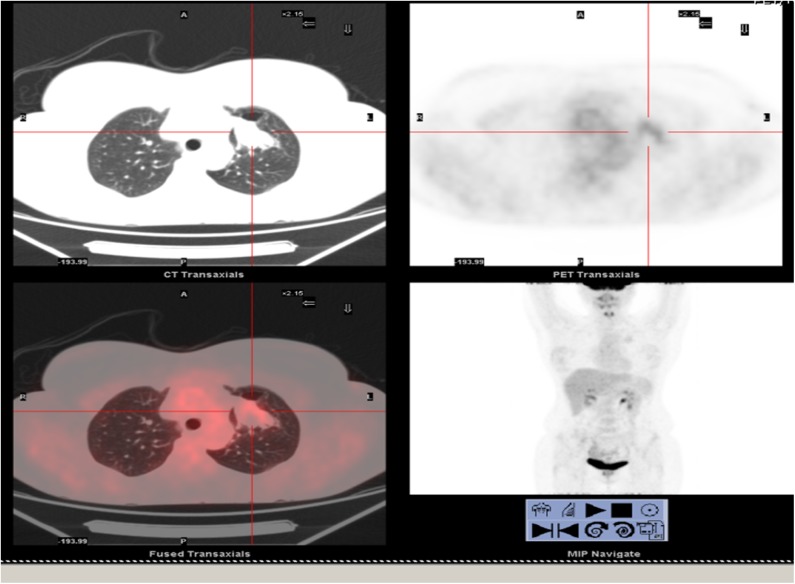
Total body PET/CT scan. Lung nodule at the upper left lobe showed a very low uptake of the lesion.
